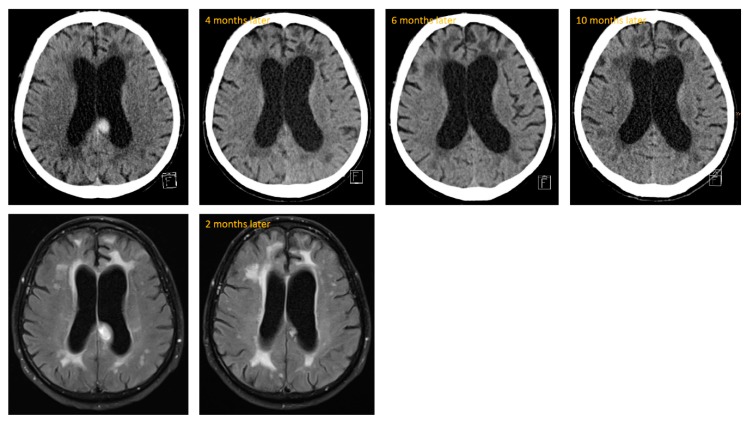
Long-term follow-up on CT (line above) and short-term follow-up on MRI (below).
